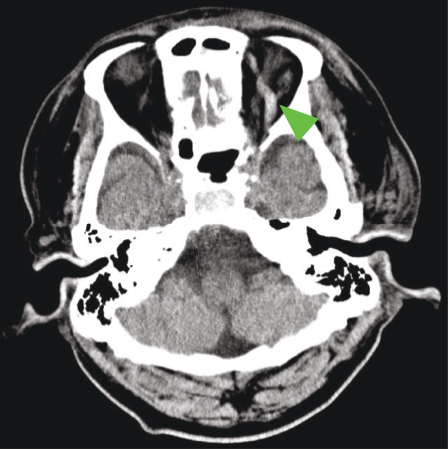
Follow-up plain computed tomography scan conducted on day 3 to screen for head injuries. The *yellow-green arrowhead* indicates the dilated left superior ophthalmic vein (to 4.8 mm)
